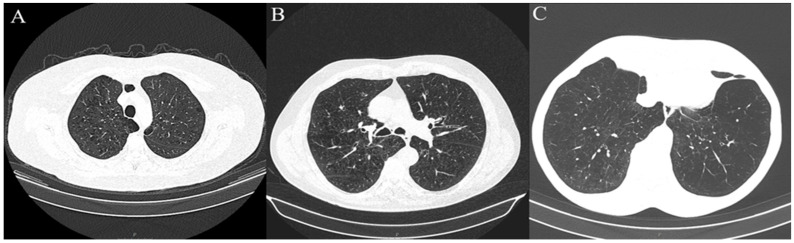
Axial chest CT scans of included patients demonstrating different emphysema types according to Fleischner guidelines. (A) Moderate centrilobular emphysema located in upper lung zone of lung parenchyma. (B) Confluent centrilobular emphysema located in middle lung zone of lung parenchyma. (C) Advanced destructive centrilobular emphysema located in lower lung zone of lung parenchyma. Patients (B,C) also have mild pareseptal and substantial paraseptal emphysema, respectively.
